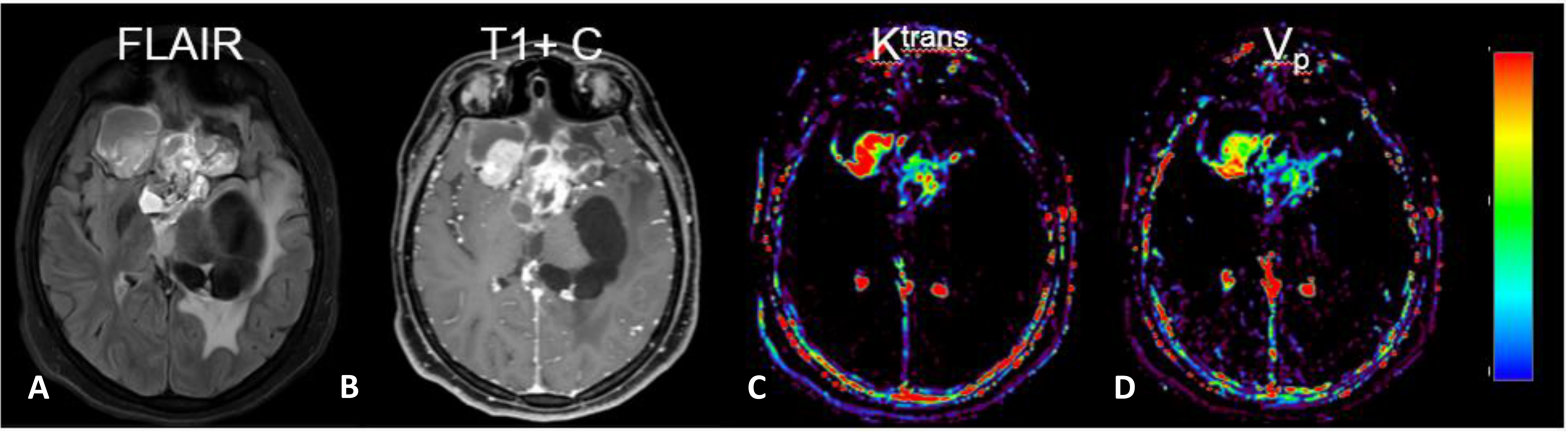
MRI with DCE parameter maps. (A) FLAIR and (B) post-contrast *T*_1_-weighted (*T*_1_+C) images of a patient with a primary brain tumor. In addition, the extended Tofts’ model was used to fit DCE-MRI data to generate parameter maps of (C) K^trans^ and (D) V_p_. (Images are courtesy of Imaging Biometrics LCC).
